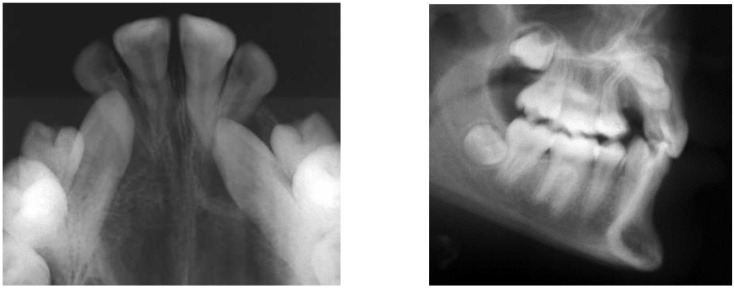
Occlusal X-ray and lateral teleradiograph corresponding to the patient in Figure 1.
